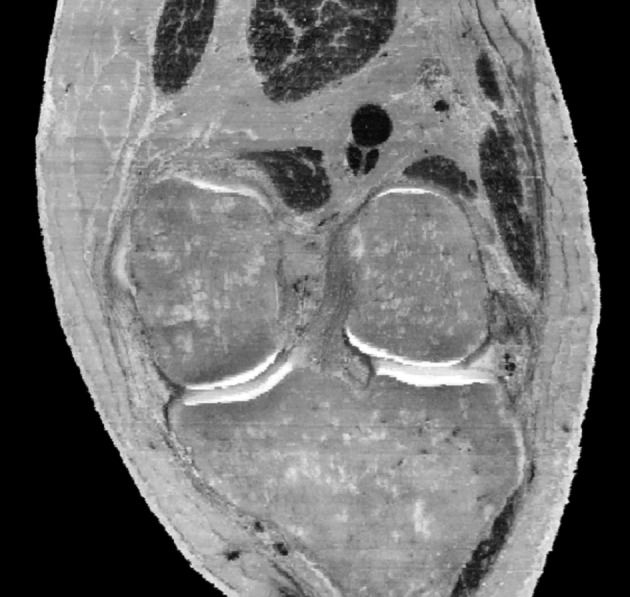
Oblique coronal plane magnetic resonance imaging (MRI) showing the posterolateral (PL) bundle.
